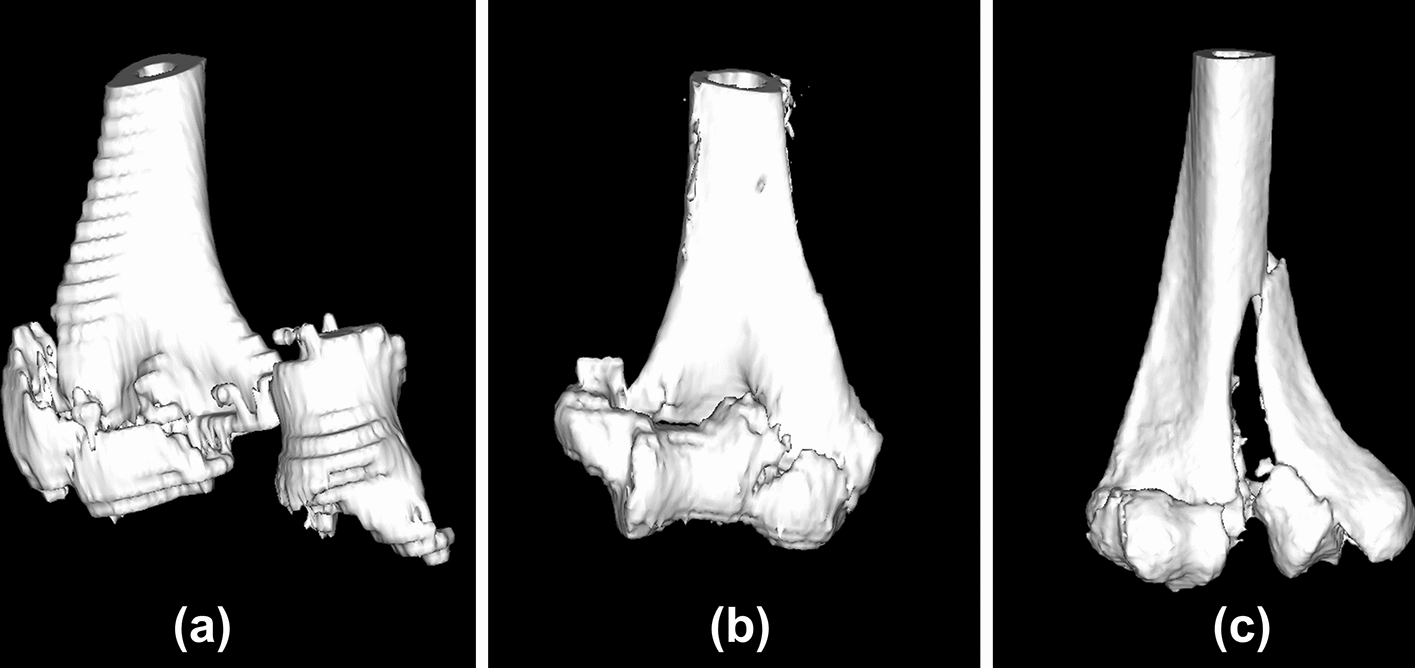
Comparison of 3D images created from CT images with different slice widths. a 3-mm slice width, b 2-mm slice width, c 1-mm slice width
